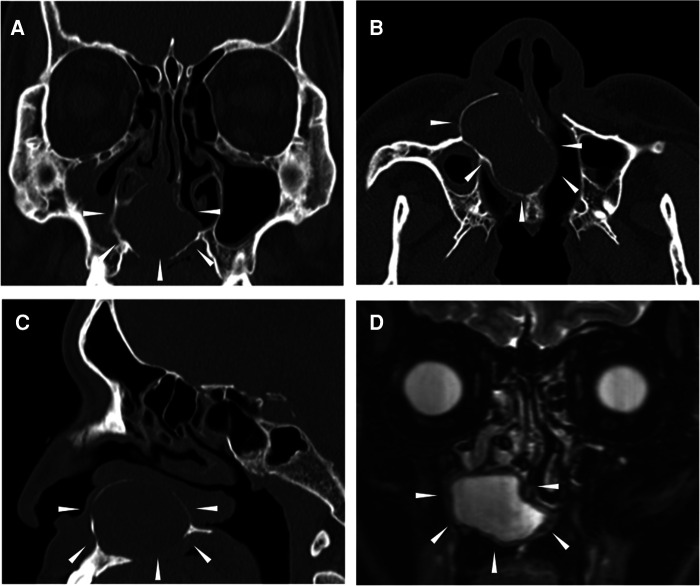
Preoperative CT scans and T2-weighted MRI of the sinuses. The area surrounded by the arrowhead indicates the lesion. (A) Coronal CT, (B) Axial CT, (C) Sagittal CT, and (D) T2-weighted MRI. CT, computed tomography; MRI, magnetic resonance image.
